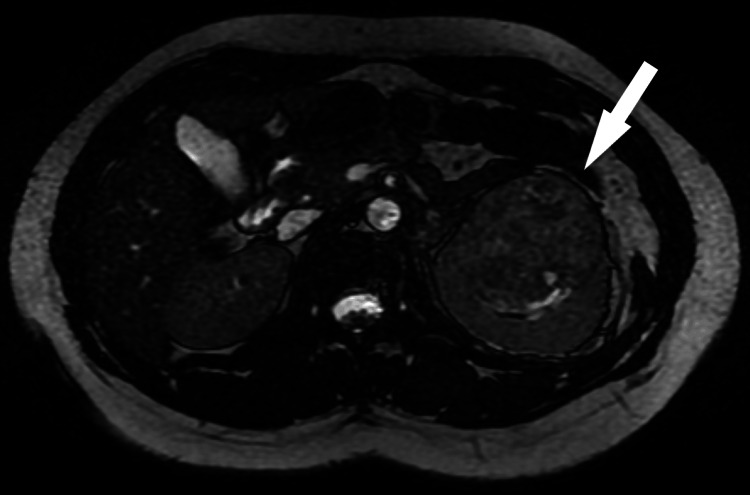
Magnetic resonance imaging of the abdomen showing the renal carcinoma (arrow)
